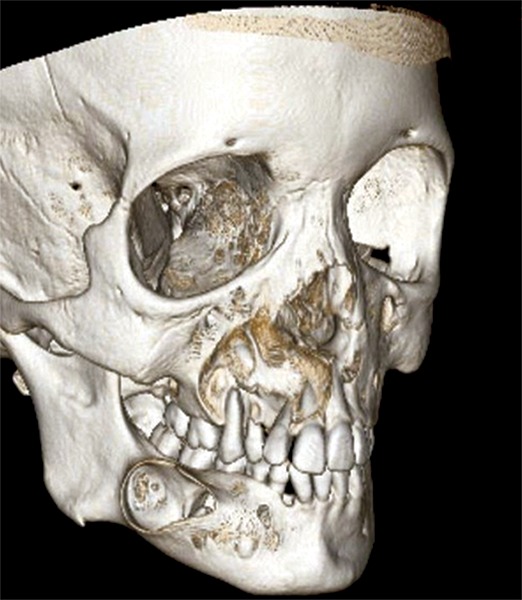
3DCT facial bones showing right lateral view of maxilla and mandible with cortical perforation involving periapical area of 12,14,15 and right maxillary sinus
